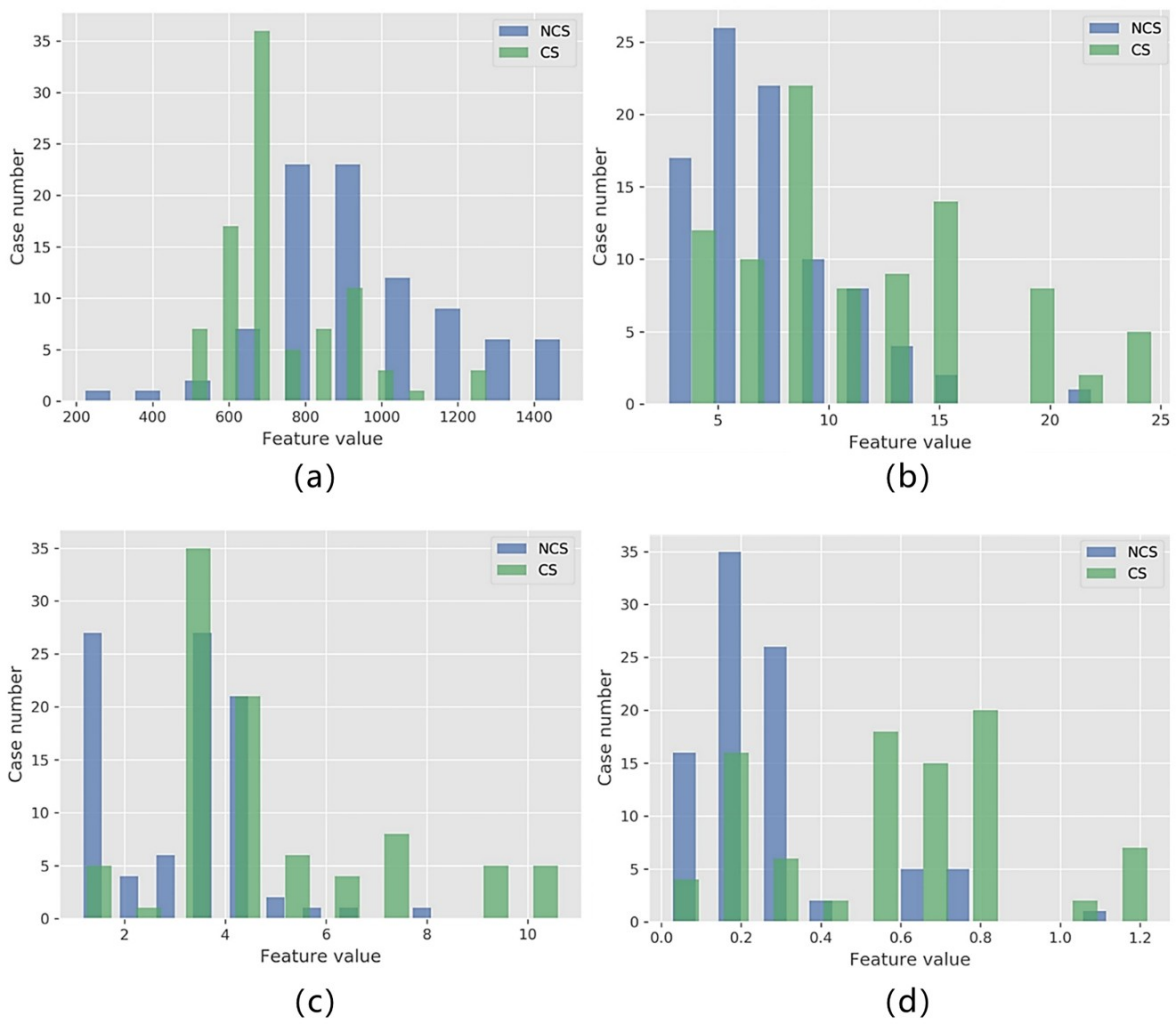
Histograms of selected features in CS PCa and NCS PCa. Distribution of *GLV*_*DWI*_, *IR*_*DWI*_, *AC*_*DWI*_, and 10*Per*_*ADC*_ shown as (a) through (d), respectively.
